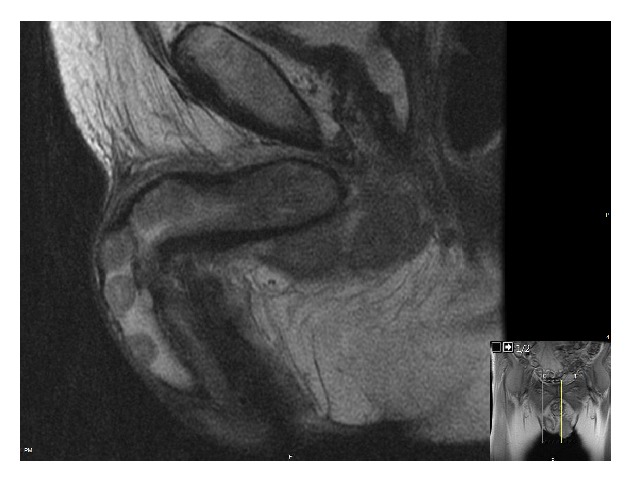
MRI showing low signal lesions invading the corpora cavernosa.
